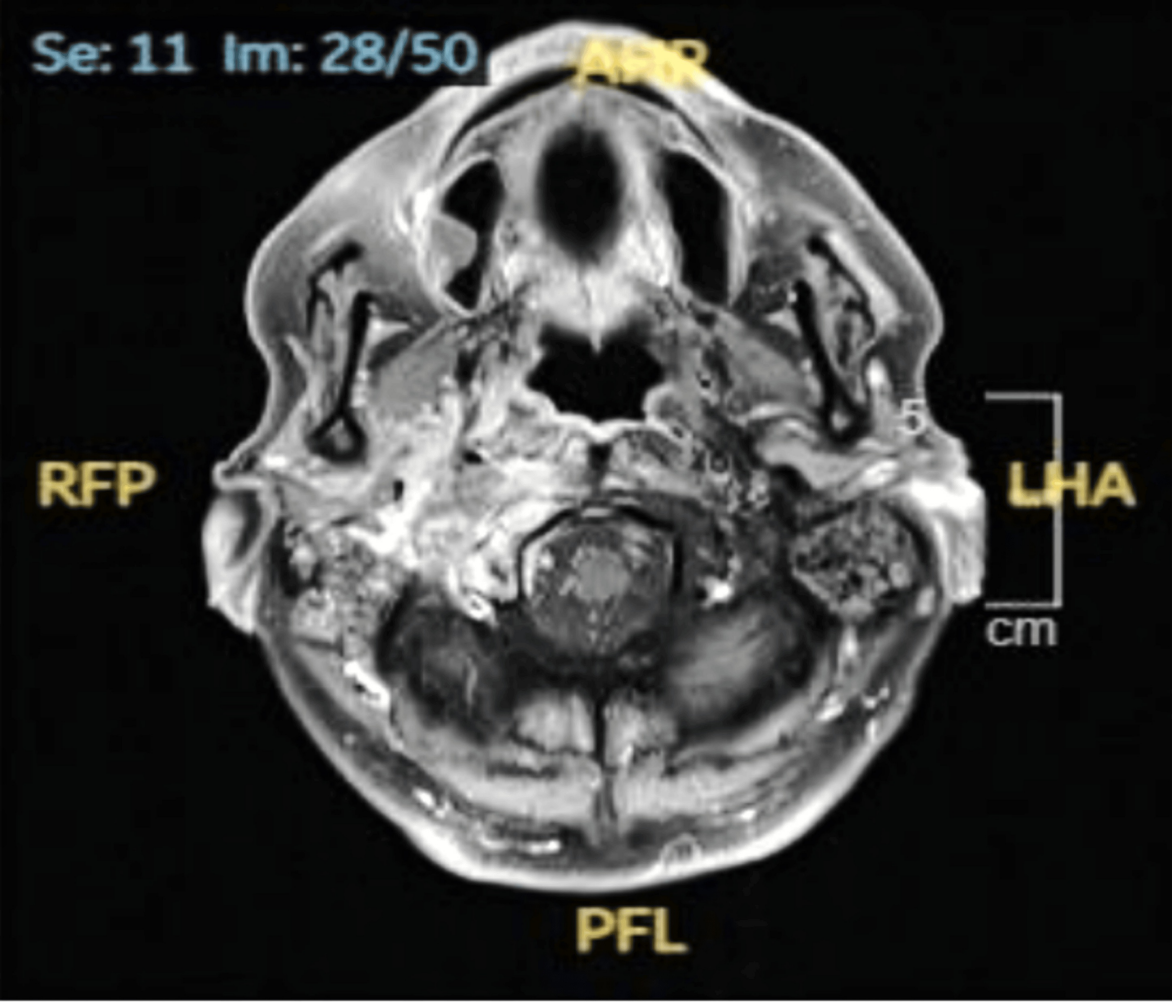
MRI of the head with contrast showing features of necrotizing external otitis on the right, with skull base osseous destruction/osteomyelitis, small mastoidal abscesses, and mastoiditis.
